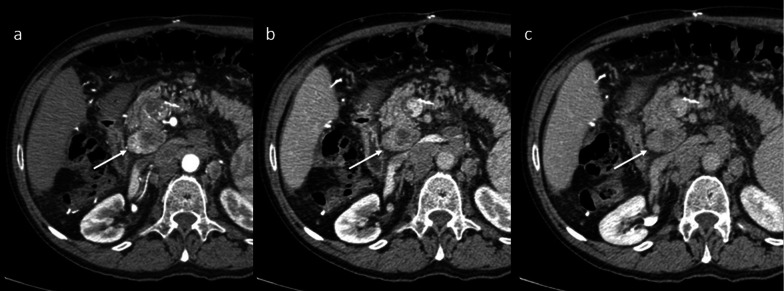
Lymph node recurrence. Axial contrast-enhanced CT images on the arterial phase (a), portal venous phase (b), and 3-min late phase (c) exhibit a metastatic portacaval lymph node with a central necrotic area, showing the typical pattern of HCC: inhomogeneous enhancement on the arterial phase and washout in the 3-min late phase
